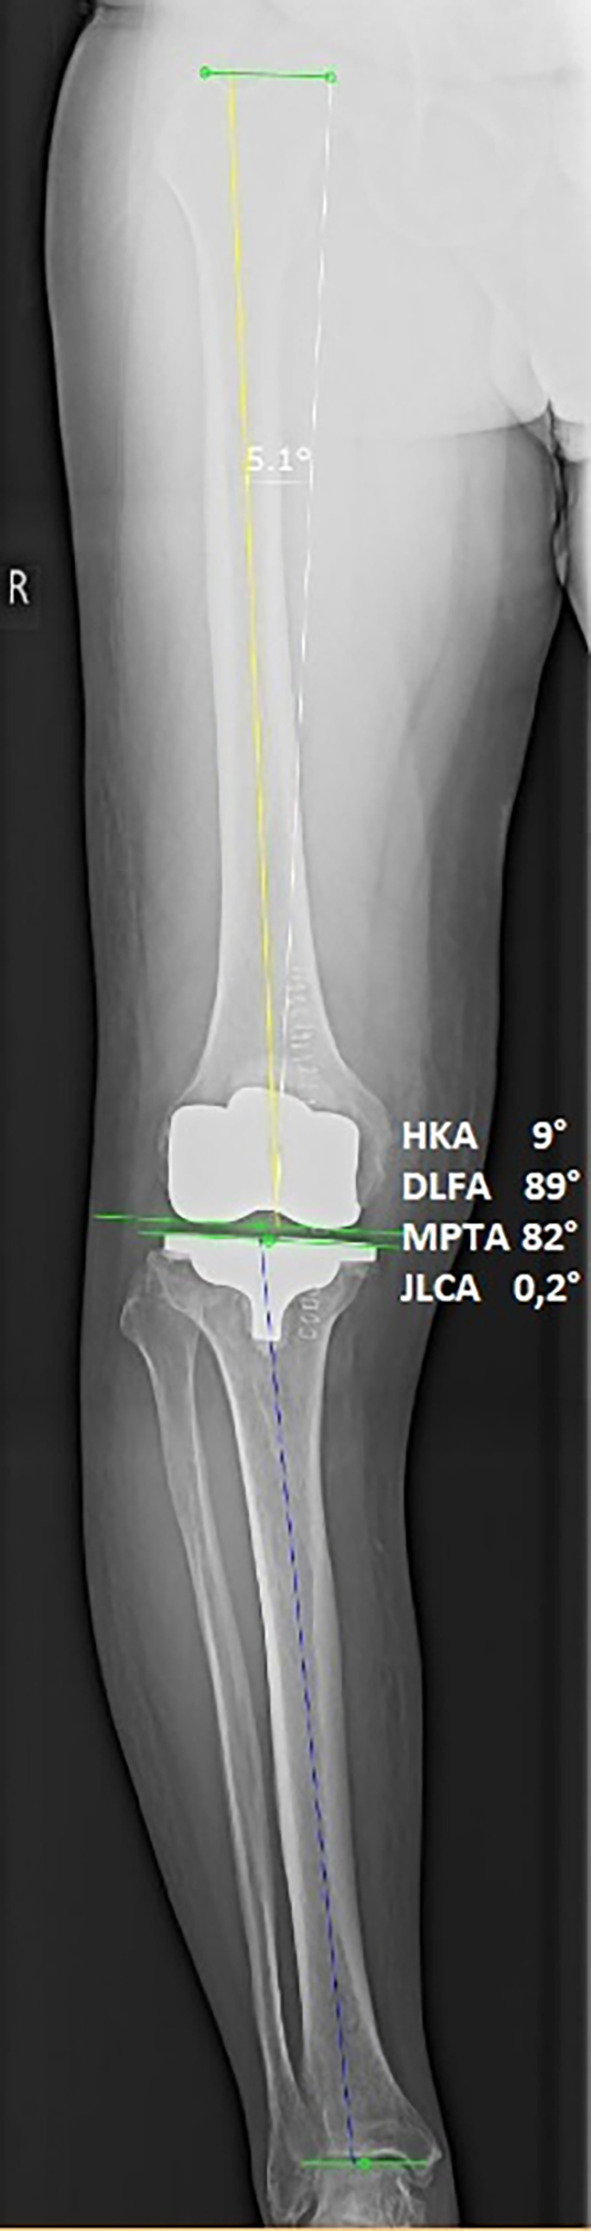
Postoperative full weightbearing long-standing anteroposterior radiograph of the knee in Figure 2 with alignment references. HKA, hip-knee-ankle angle; JLCA, joint line convergence angle; LDFA, lateral distal femural angle; MPTA, medial proximal tibial angle.
